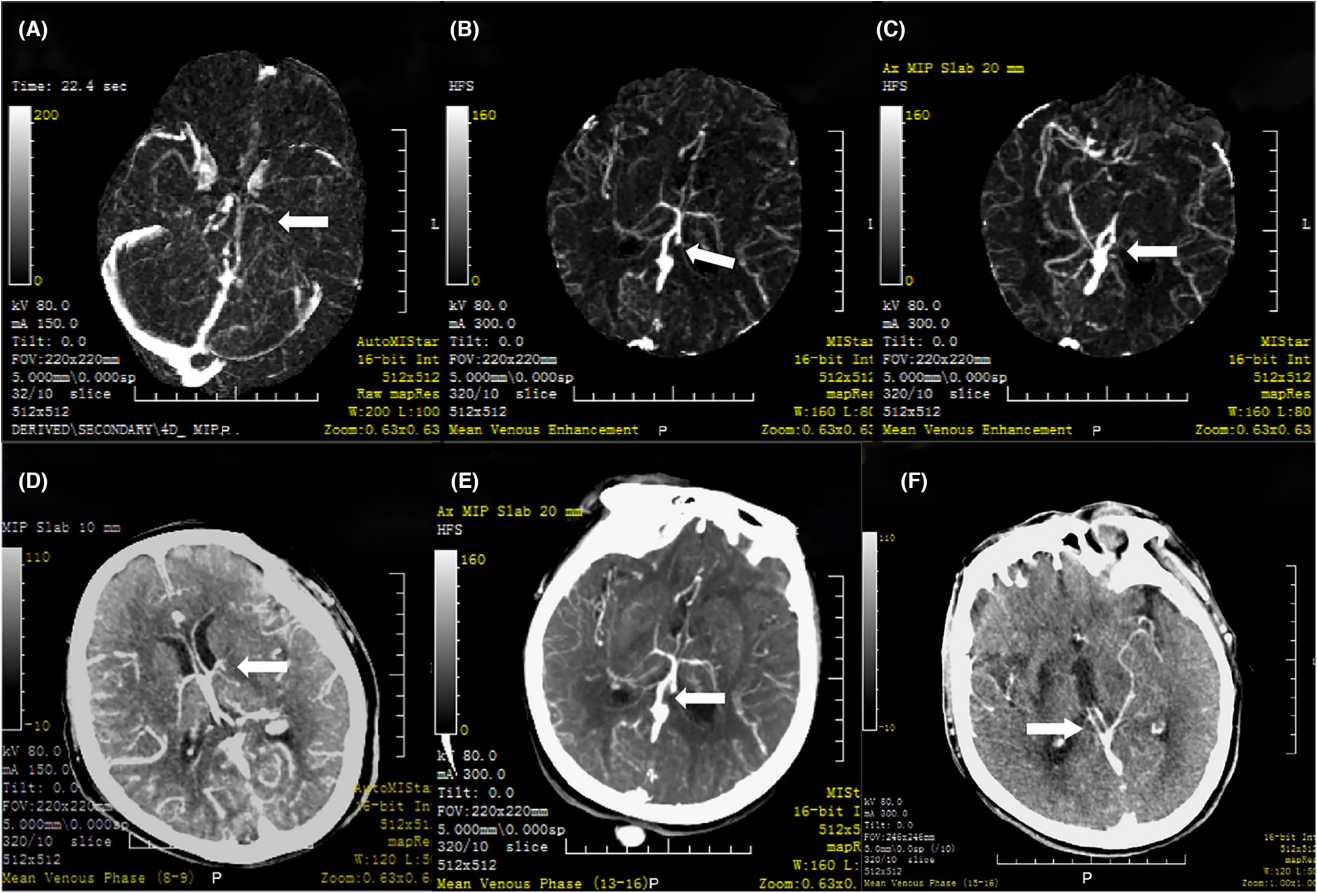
Observation and evaluation of deep cerebral venous filling. Deep cerebral venous filling can be observed on both the reconstructed 4D‐DSA and the mean venous map derived from CT perfusion software. (A–C) represent the asymmetric thalamostriate vein (TSV), internal cerebral vein (ICV), and basal vein of Rosenthal (BVR) observed on the reconstructed 4D‐DSA map, while (D–F) represent the asymmetric TSV, ICV, and BVR observed on the mean venous map.
